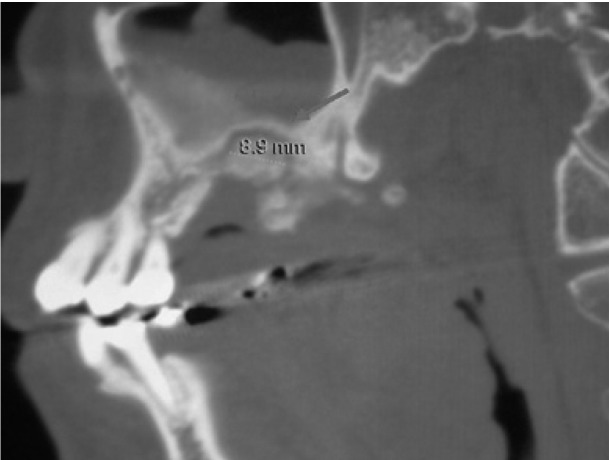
CT image demonstrating bone sequestrum of 8.9 mm diameter in the affected area (red arrow).
